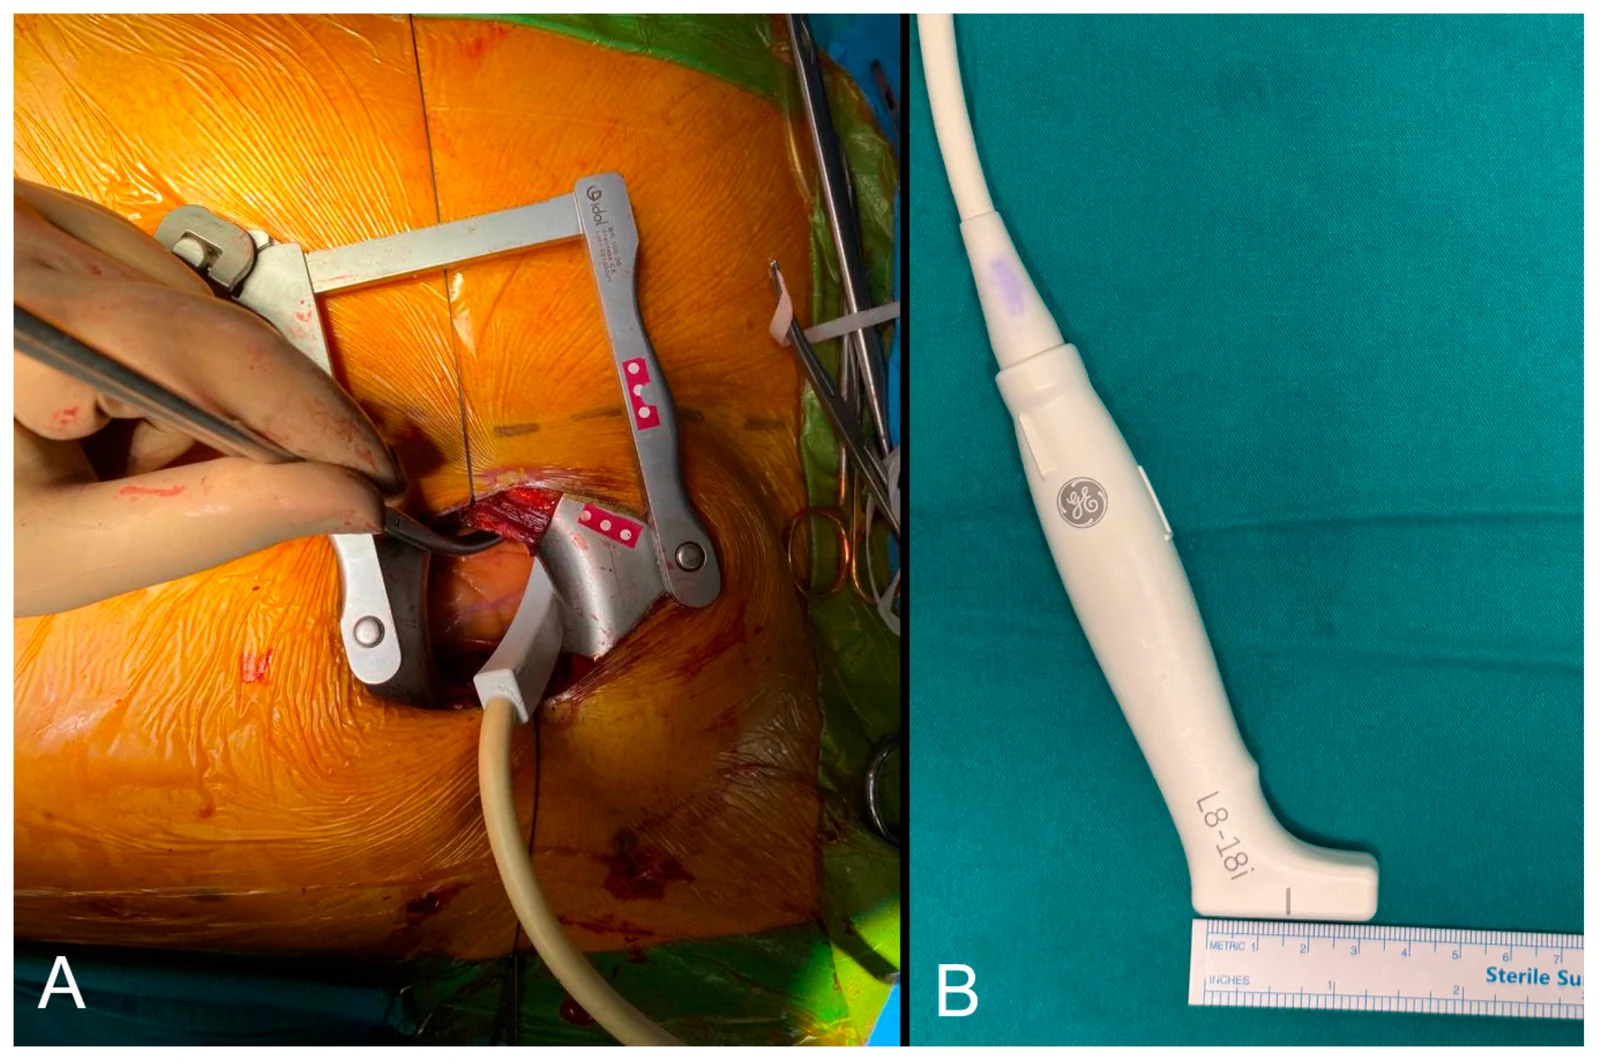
(A) The epiaortic ultrasound probe can be easily introduced through the minithoracotomy. (B) The “hockey-stick” probe (L8-18i-RS linear array transducer, GE HealthCare, Chicago, IL, USA) used for epiaortic ultrasonography.
